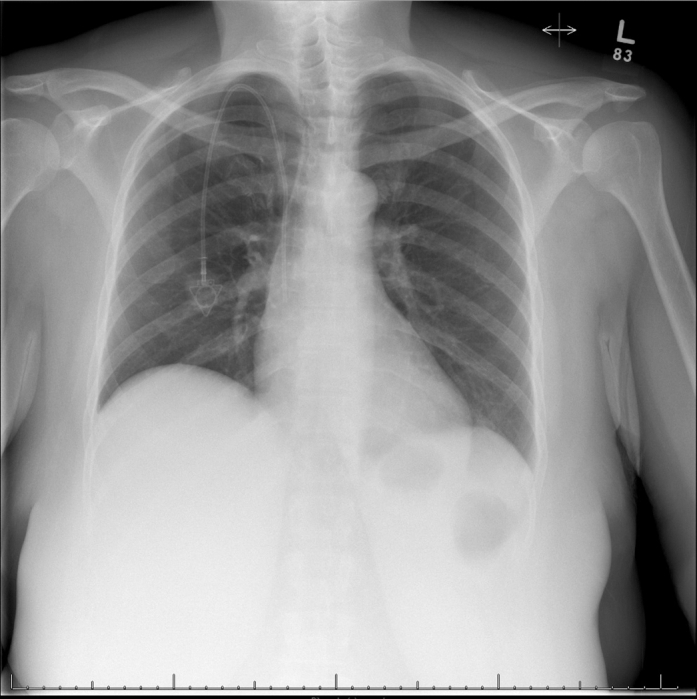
Baseline Anteroposterior Chest Radiograph Frontal chest radiograph on presentation, showing clear lung fields without consolidation or interstitial edema.
